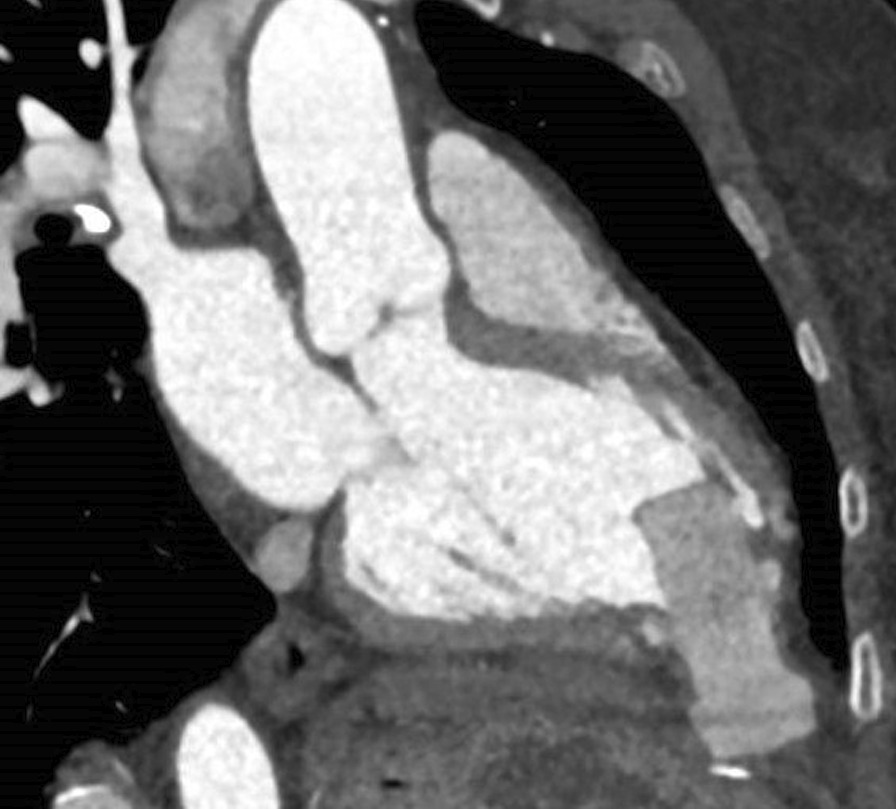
Case 10: Figure 4. Chest CT coronal plane. LV assist device felt plug in the LV apex with no metal present
